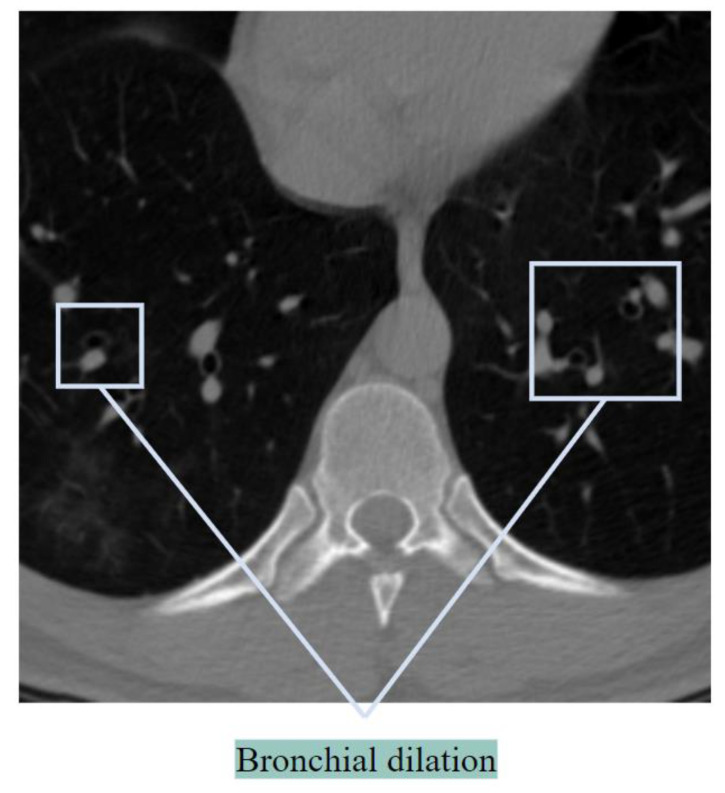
Bronchial dilation showed in a CT scan.
